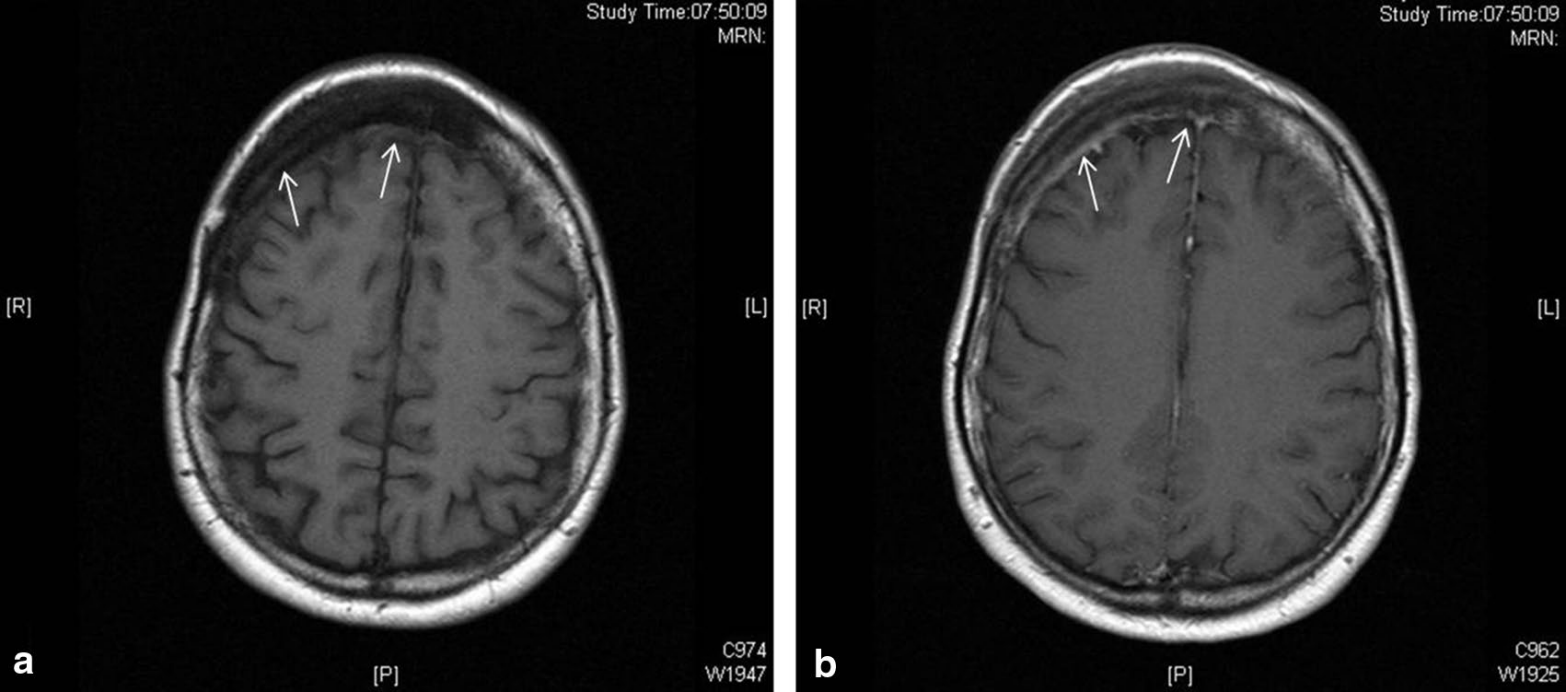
a Axial T1-weighted MR image shows thickening of the right frontal part of the diploe (*arrows*) with an underlying extensive low signal osseous lesion. b Axial T1-weighted MR image post gadolinium shows minimal enhancement of the periosteal part of the lesion (*arrows*). There is no evidence of abnormality related to the brain parenchyma
